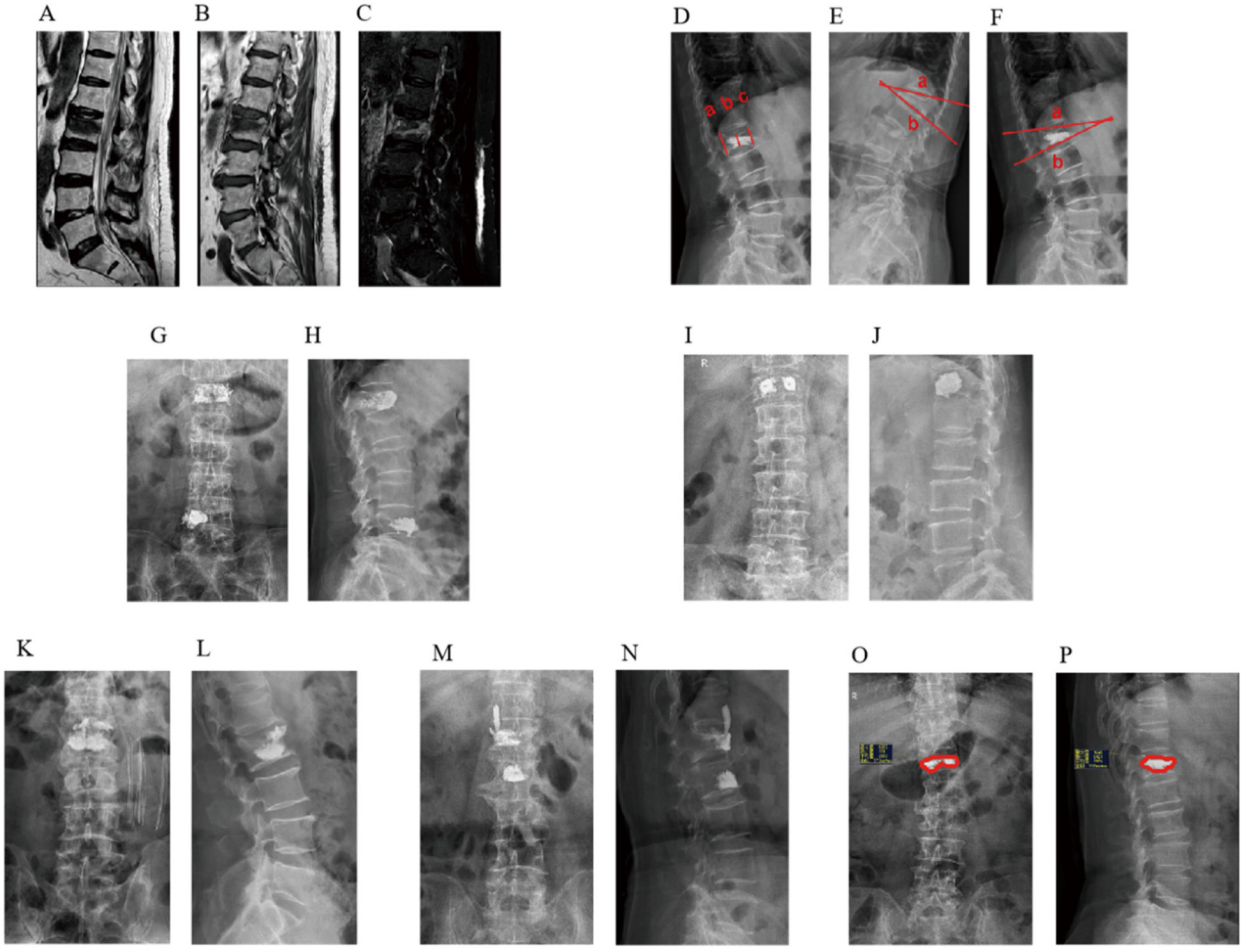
MRI findings of fascial edema after osteoporotic vertebral compression fracture. (A) T1WI, (B) T2WI, (C) T2-STIR WI; Imaging evaluation of the vertebral body of osteoporotic vertebral compression fracture. (D) Anterior vertebral height (AVH), Middle vertebral height (MVH), Postoperative Posterior vertebral height (PVH), (E) Preoperative Cobb angle, (F) Postoperative Cobb angle; Distribution characteristics of spongy bone cement. (G) anteroposterior radiograph of spongy diffuse distribution pattern, (H) lateral radiograph of spongy diffuse distribution pattern; Distribution characteristics of massive bone cement. (I) anteroposterior radiograph film of the local solid distribution pattern of massive bone cement, (J) lateral radiograph of massive local solid distribution pattern; Image of bone cement leaking into the intervertebral space. (K) anteroposterior radiograph of bone cement leaking into the intervertebral space, (L) lateral radiograph of bone cement leaking into the intervertebral space; Image of bone cement leaking into the paravertebral tissue. (M) anteroposterior radiograph of bone cement leaking into the paravertebral tissue, (N) lateral x-ray film of bone cement leaking into paravertebral tissue; Bone cement perfusion ratio. (O) coronal bone cement perfusion ratio, (P) sagittal bone cement perfusion ratio.
